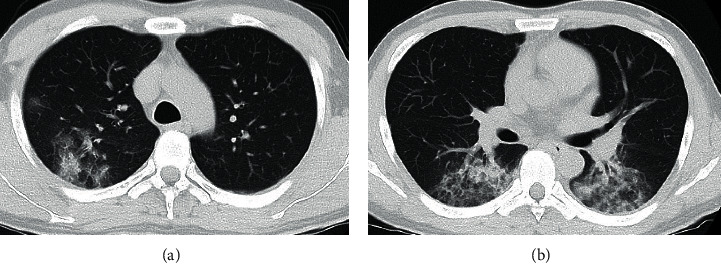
Preoperative chest computed tomography (CT) radiological findings. A chest axial CT in the lung window setting showed a consolidation and ground-glass opacity in the posterior segments of the right upper lobes (a) and the superior segments of the bilateral lower lobes (b), consistent with aspiration pneumonia.
